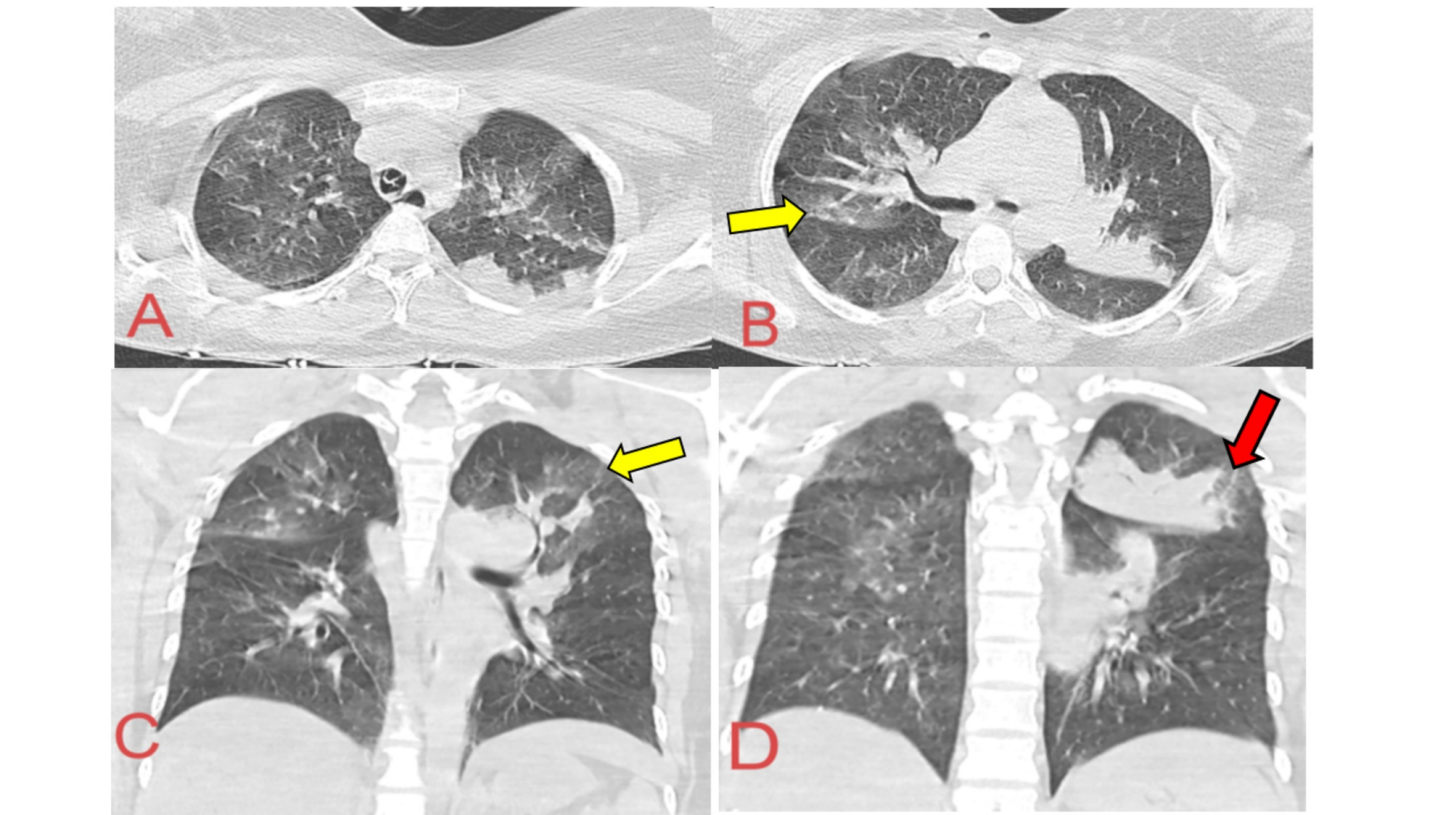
CT chest images of cardiac arrest in a young female patient. A&B: Axial views showing bilateral mosaic changes and peripheral ground glass opacities (GGOs) in both upper lobes (yellow arrows) with consolidation of the left upper lobe (red arrow). C&D: Coronal views confirming the same findings.
